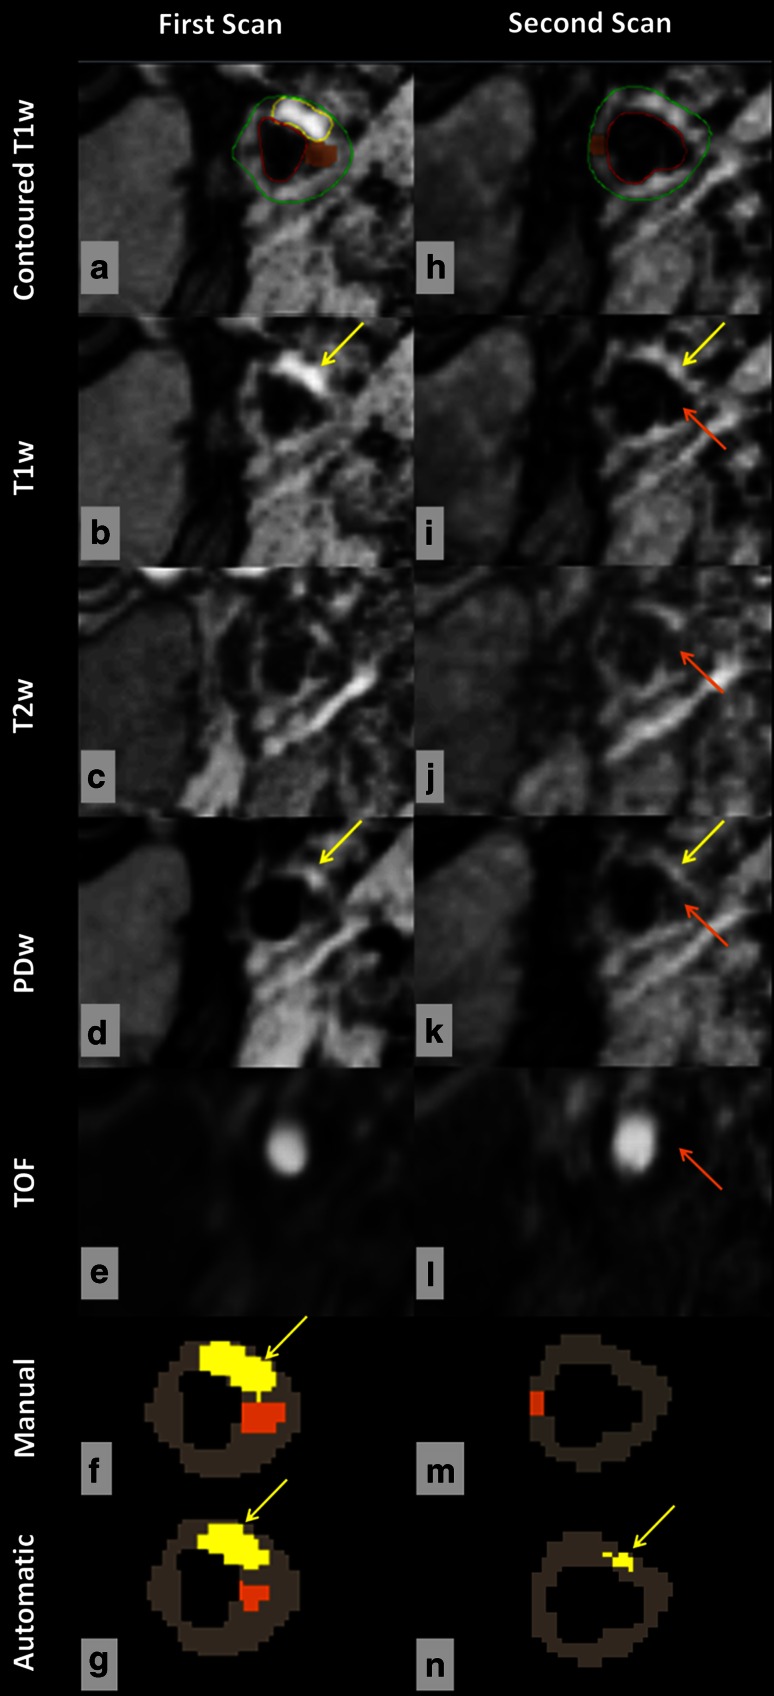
Manual and automated classification of lipid and calcification using multicontrast MR images obtained in repeated scans. Manual contours overlaid on the T1w image (a, h). Lipid = *yellow*, calcification = *orange*, lumen = *red*, outer wall = *green*. Using SCM muscle as reference, lipid (*yellow arrow*) appears hyperintense on T1w and PDw images. As can be seen, the classifier consistently identifies lipid (*yellow region*) in two scanning sessions (g, n), while the observer does not (f, m). Both segmentation methods could identify calcification (*orange region*) in the first scan (f, g), but no calcification was found at the same location in the second scan (m, n) because the observer annotated the region of juxta-luminal calcification (*orange arrow*) as lumen
